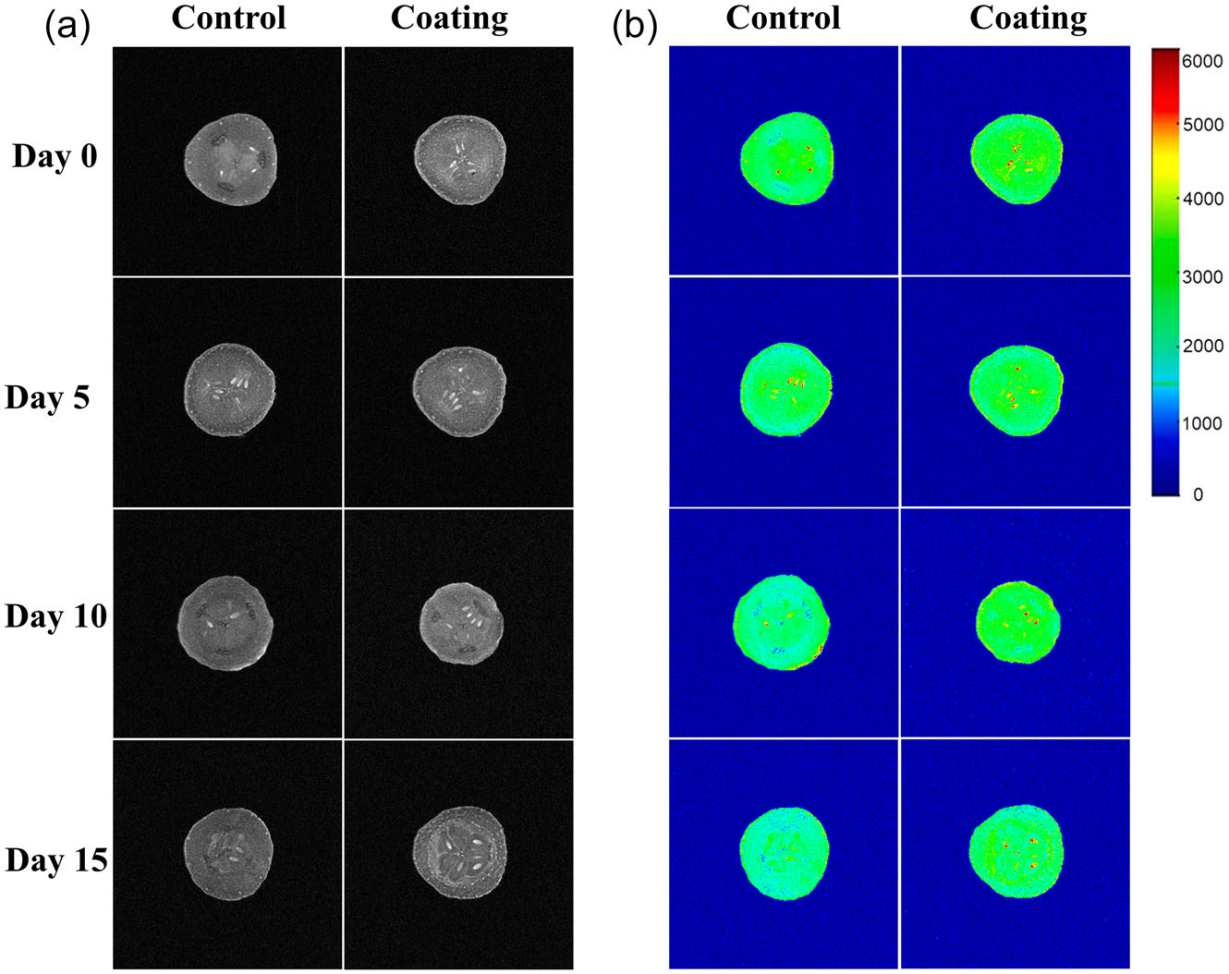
The magnetic resonance imaging (MRI) gray‐scale image (a) and pseudo‐color image (b) of cucumbers during cold storage.
